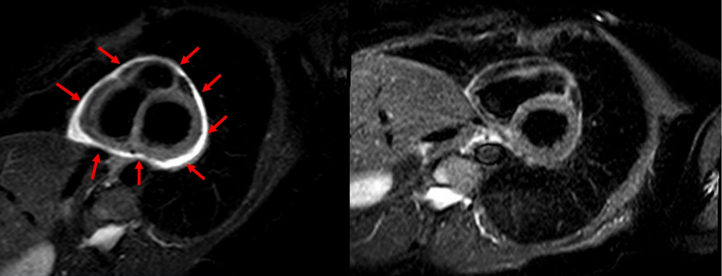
Case Presentation #4 A 66-year-old female who developed recurrent pericarditis after a coronary perforation during a percutaneous coronary intervention of her left anterior descending artery. The image on the left is a short-axis slice of cardiac magnetic resonance imaging showing late gadolinium enhancement, edema, and pericardial thickening (arrows). The image on the right is repeat imaging of the same patient 1 year later after completion of treatment with aspirin, colchicine, and prednisone noting significant improvement.
